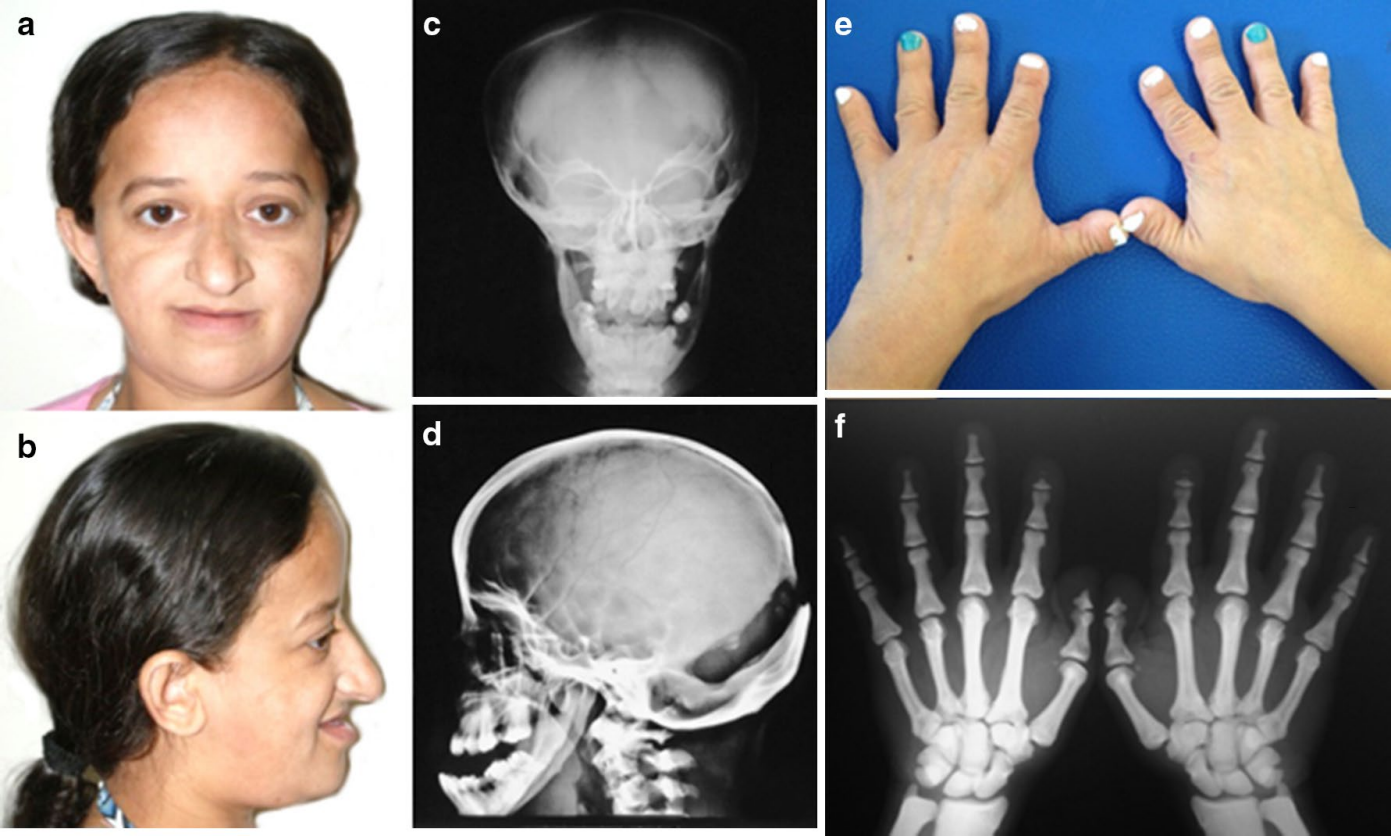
Clinical and radiological findings of two patients affected by pycnodysostosis. a–b The typical facial appearance of the pycnodysostosis (large frontal, midfacial hypoplasia, low set ears, and beaked nose), here observed in the patient 27. c–d Cranial X-rays of patient 27 showing increased bone density, opened sutures, parietal bossing and obtuse mandibular angle. e The hands of patient 27 showing brachydactyly. f X-rays of the hands of the patient 2 showing increased bone density, brachydactyly and acroosteolysis of the distal phalanges, mainly observed in both second fingers
